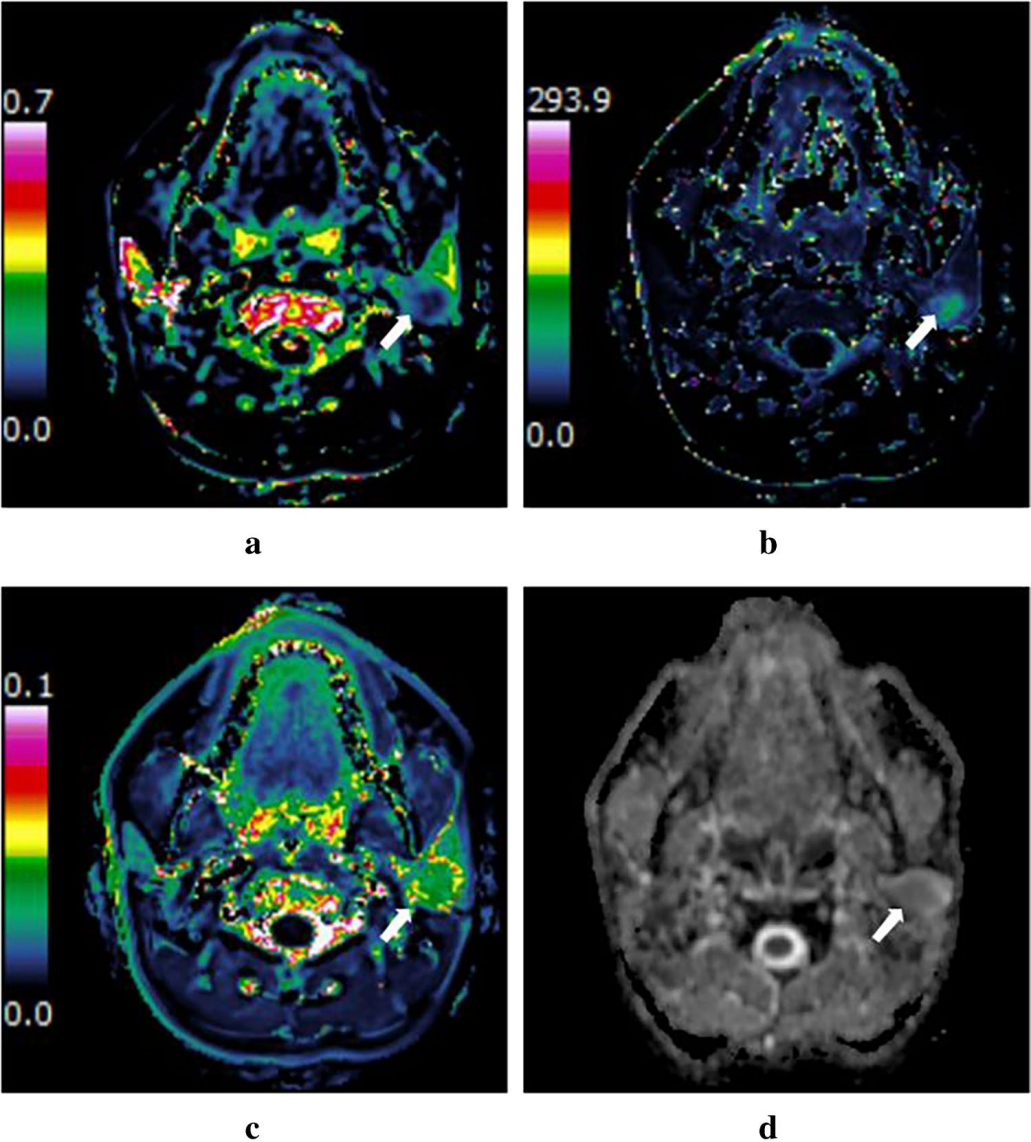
A 34-year-old man with pleomorphic adenoma in the left parotid gland (white arrow). Dynamic contrast-enhanced MRI parameter maps showed the *K*_ep_ (a), *V*_e_ (b), and *K*^trans^ (c) values of 0.30 min^−1^, 0.51, and 0.15 min^−1^, respectively. d
